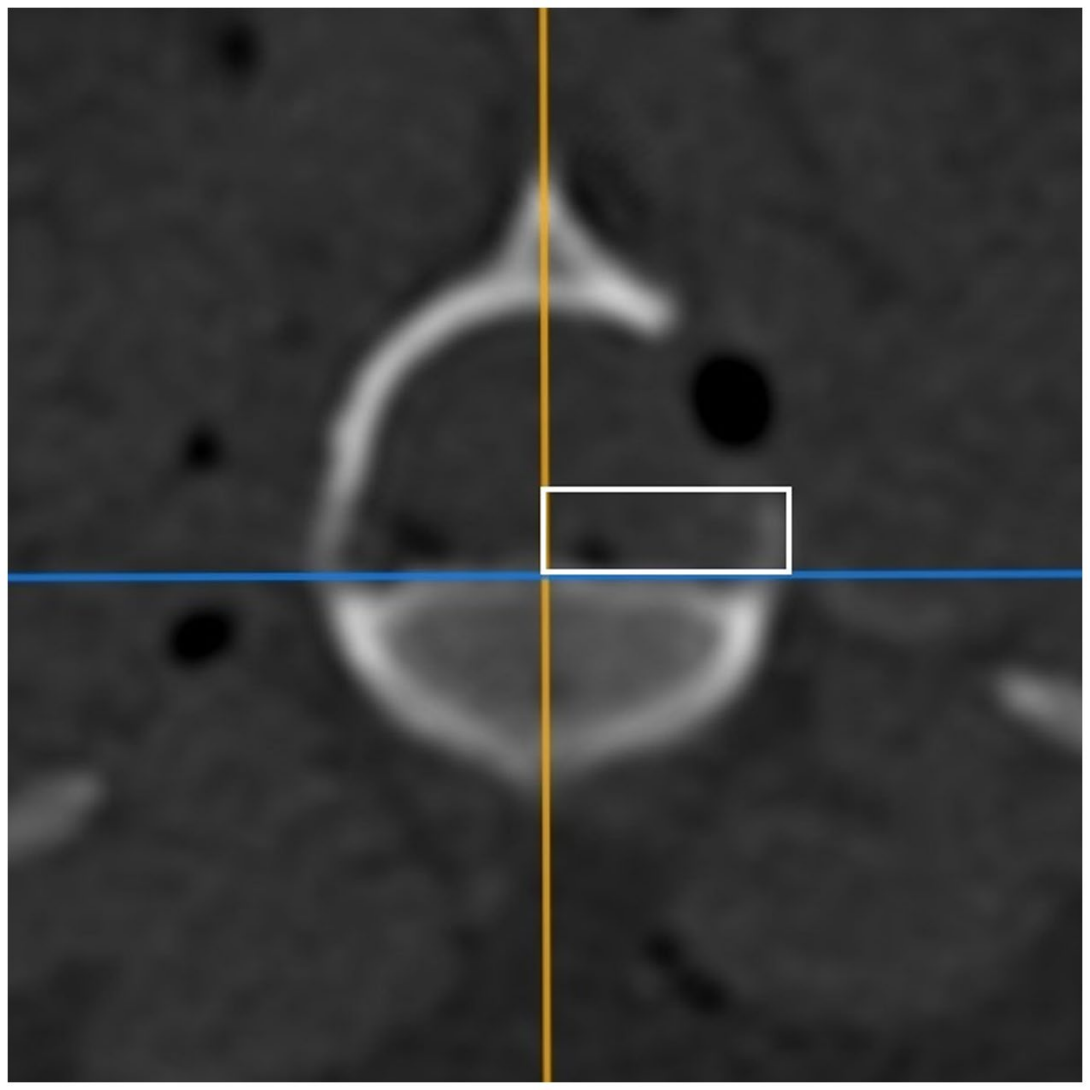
CT image in the transversal plane. The vertical line of the white rectangle represents the distance between the vertebral canal floor and the ventral border of the mini-hemilaminectomy at the level of the cranial end of the opposite foramen (ventral remnant).
